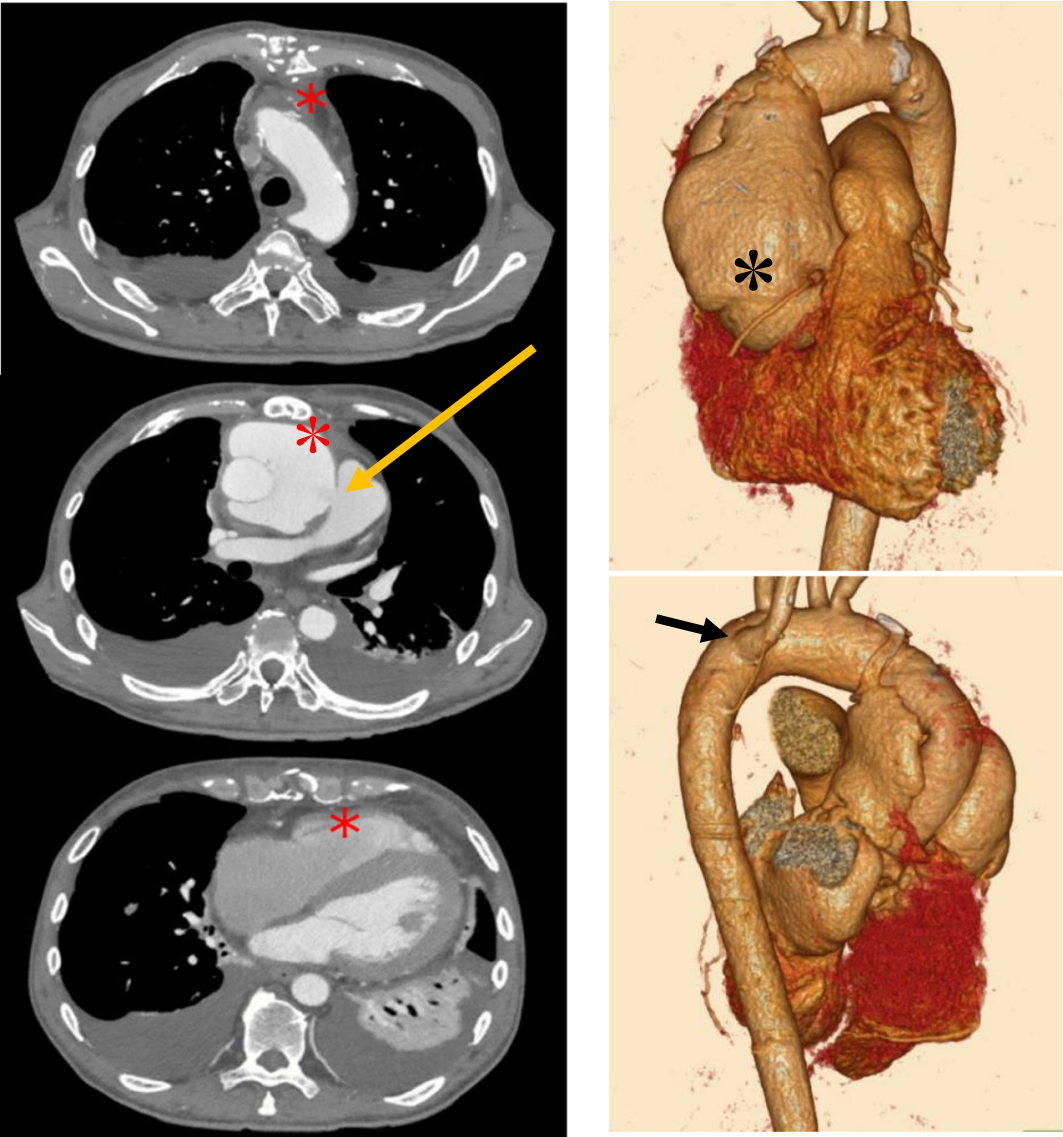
The preoperative images of transverse (left) and three-dimensional (3D) reformatted computed tomography (right). The images showed a large pseudoaneurysm (black asterisk), extensive adhesions between the aneurysm and the sternum (red asterisk), abnormal blood flow from the aneurysm to the main pulmonary artery (yellow arrow), and angiectopia of the right subclavian artery originating from the distal aortic arch (black arrow)
